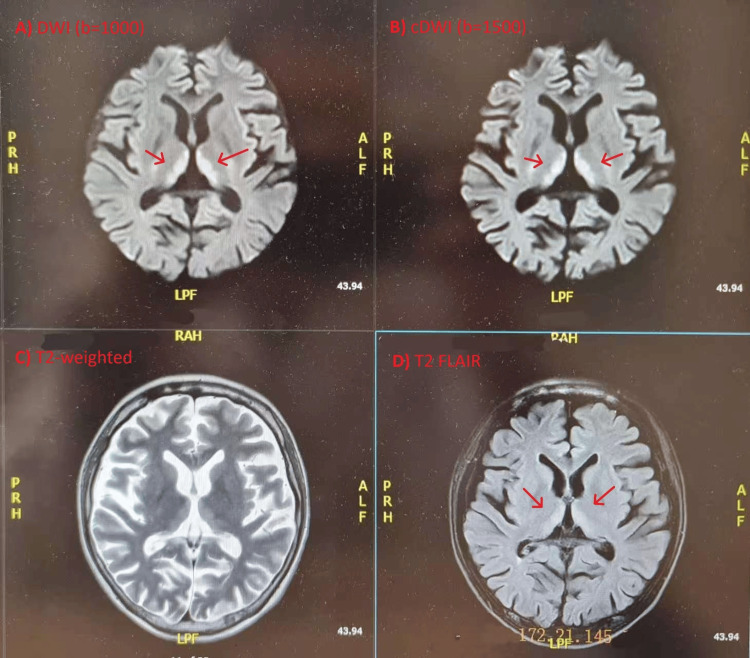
Brain MRI Axial brain MRI shows symmetric hyperintensities around the periaqueductal gray matter and medial thalami on FLAIR and diffusion-weighted imaging, consistent with Wernicke encephalopathy. FLAIR: Fluid Attenuated Inversion Recovery
